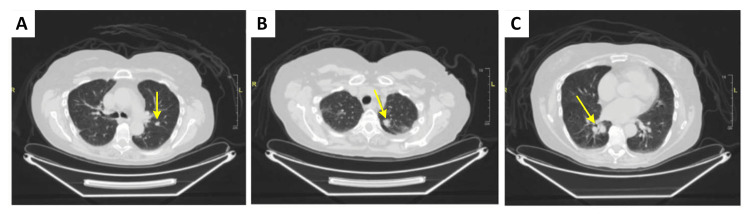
Axial chest CT images demonstrating three distinct pulmonary nodules biopsied during a single robot-assisted bronchoscopy procedure. A) Left upper lobe nodule #1, diagnosed as a typical carcinoid tumor (well-differentiated neuroendocrine tumor, grade 1). B) Left upper lobe nodule #2, diagnosed as well-differentiated pulmonary adenocarcinoma with a predominant acinar pattern. C) Right lower lobe nodule, diagnosed as poorly differentiated pulmonary adenocarcinoma with a mostly solid pattern. Yellow arrows indicate the location of each nodule.
